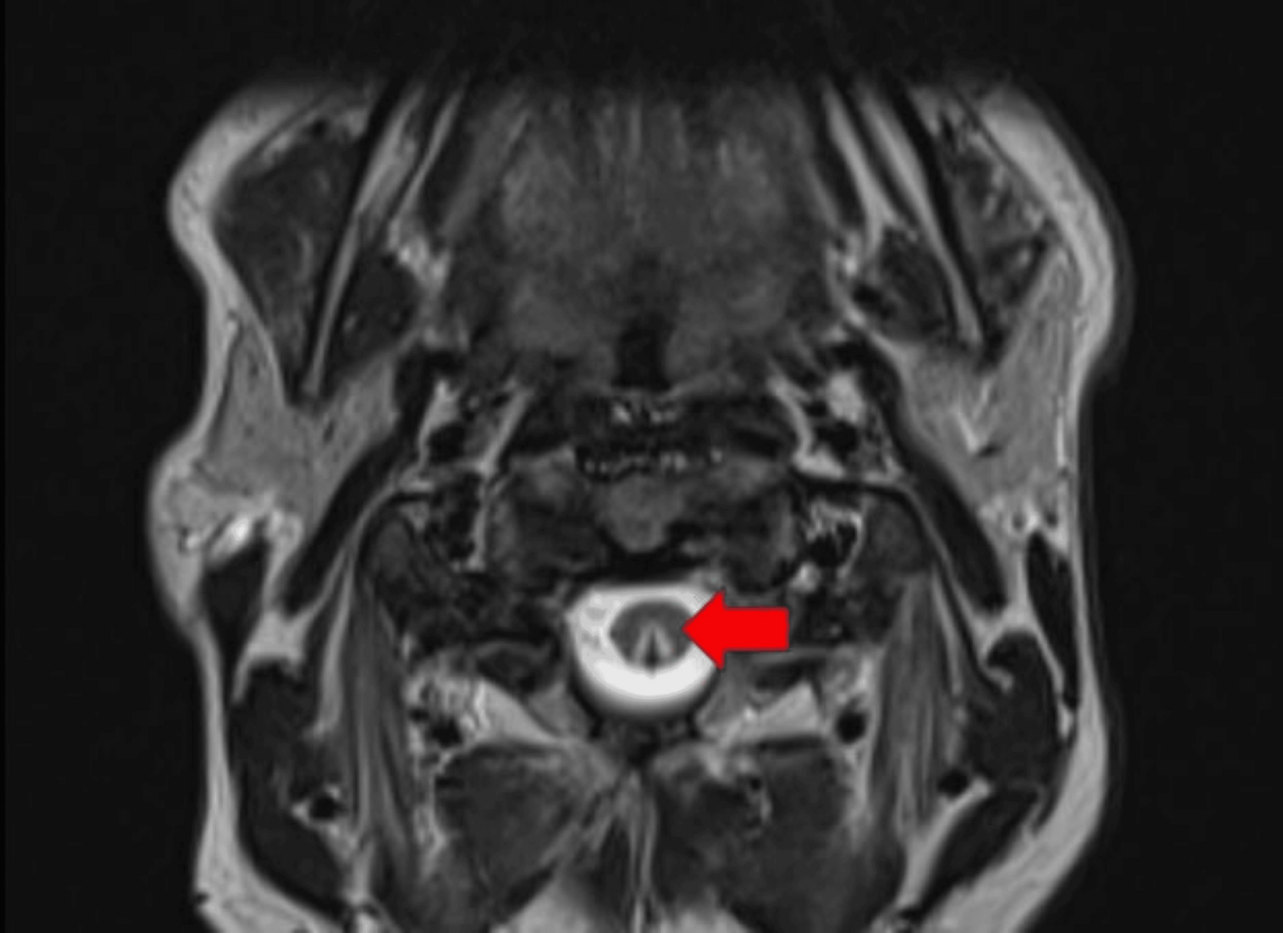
Closer axial view of signal abnormalities confined to the dorsal columns. The red arrow indicates the areas of subacute combined degeneration on MRI.
